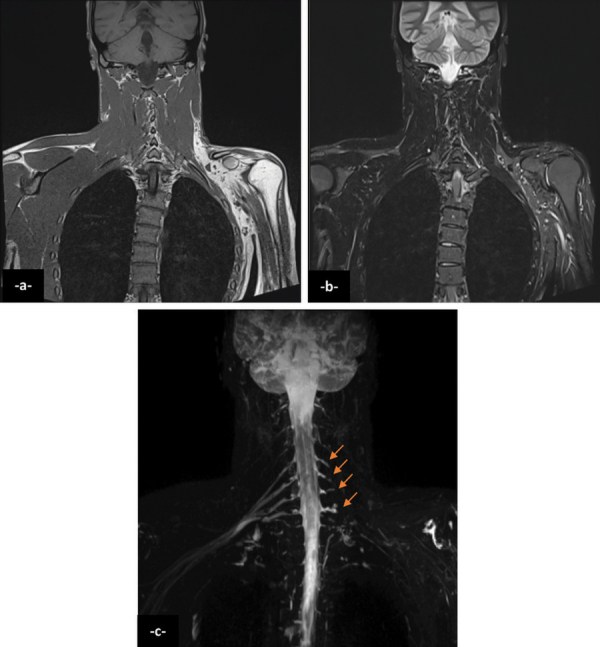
Coronal T1 and T2 FAT SAT magnetic resonance imaging (MRI) of the brachial plexus were performed, showing fatty infiltration of the left shoulder and upper limb muscles (a,b). Contrast-enhanced coronal 3D SPACE STIR showed complete discontinuity of the left brachial plexus from C4 to C7 at the level of post-ganglionic segments with retraction of distal branches (c, arrows).
